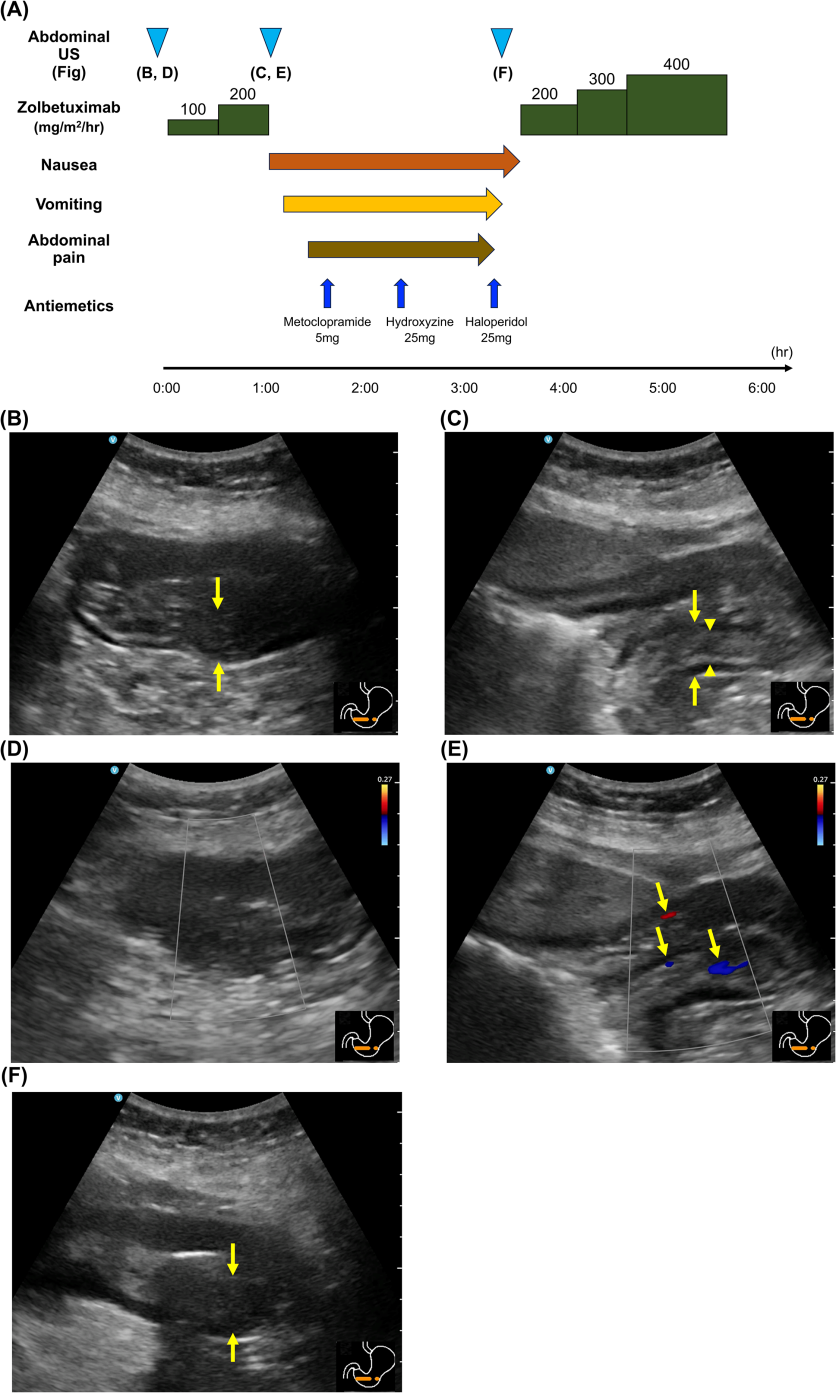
(A) Treatment course with the first cycle of zolbetuximab in Case 1. (B) Baseline ultrasound (US) showed the primary lesion of gastric cancer as a thickened hypoechoic wall with an indistinct layer structure (arrows). (C) At the onset of nausea, increased echogenicity of the submucosal layer (arrowheads) and enhanced layer stratification (arrows) were observed. (D) Color Doppler imaging at baseline revealed no detectable blood flow signals in the primary lesion. (E) At the onset of nausea, an increase in blood flow signals was observed (arrows). (F) With improvement in nausea, the echogenicity of the submucosal layer decreased, and the layer structure became indistinct again (arrows).
